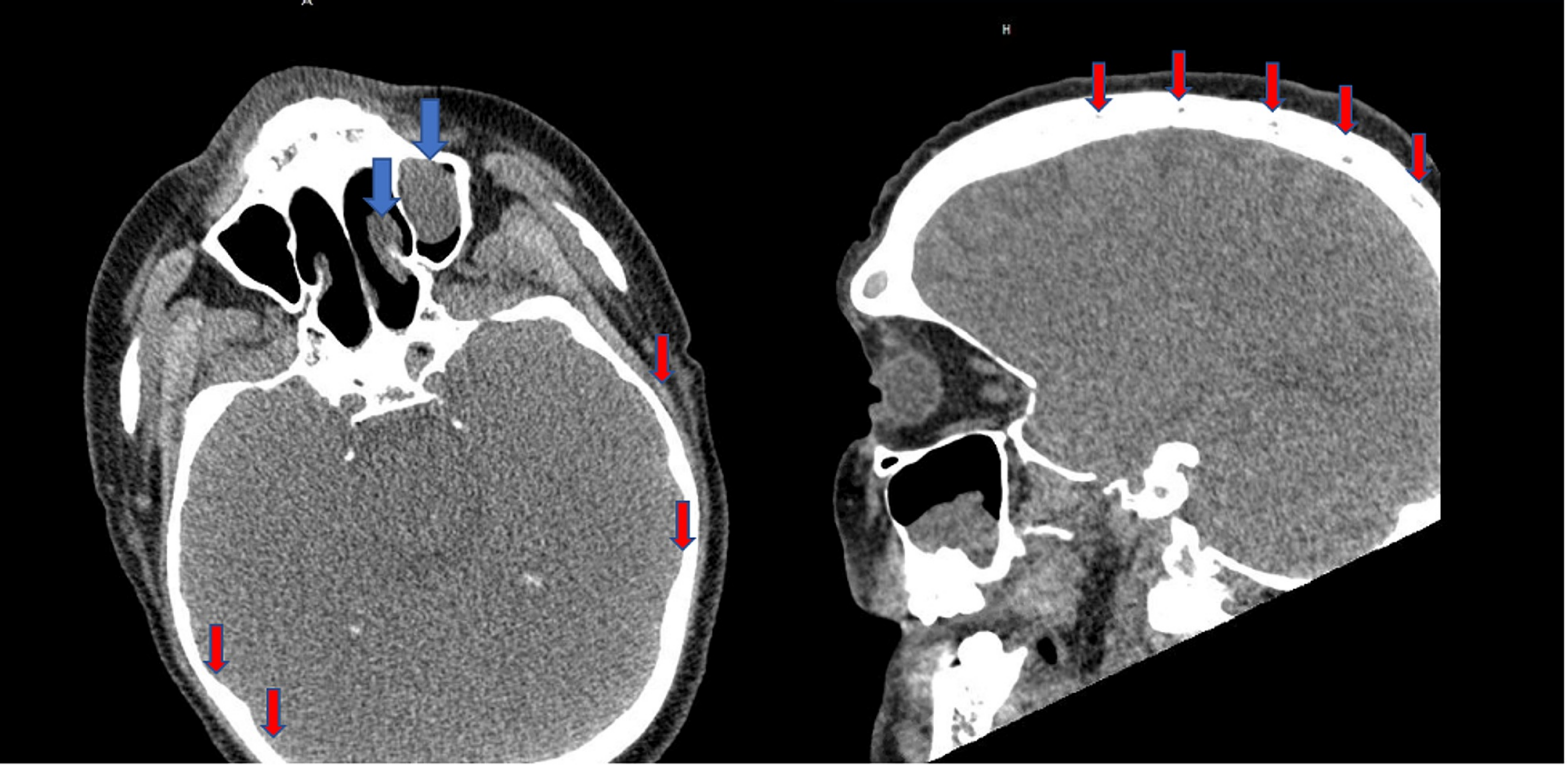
CT scan of head and sinuses. Complete opacification of the left frontal sinus and left frontal, maxillary and sphenoid sinus disease with small mucous retention cysts in the right sphenoid sinus and leftward nasal septal deviation is seen (blue arrows) and innumerable mixed lytic and sclerotic lesions within the calvarium suspicious for multiple myeloma (red arrows).
